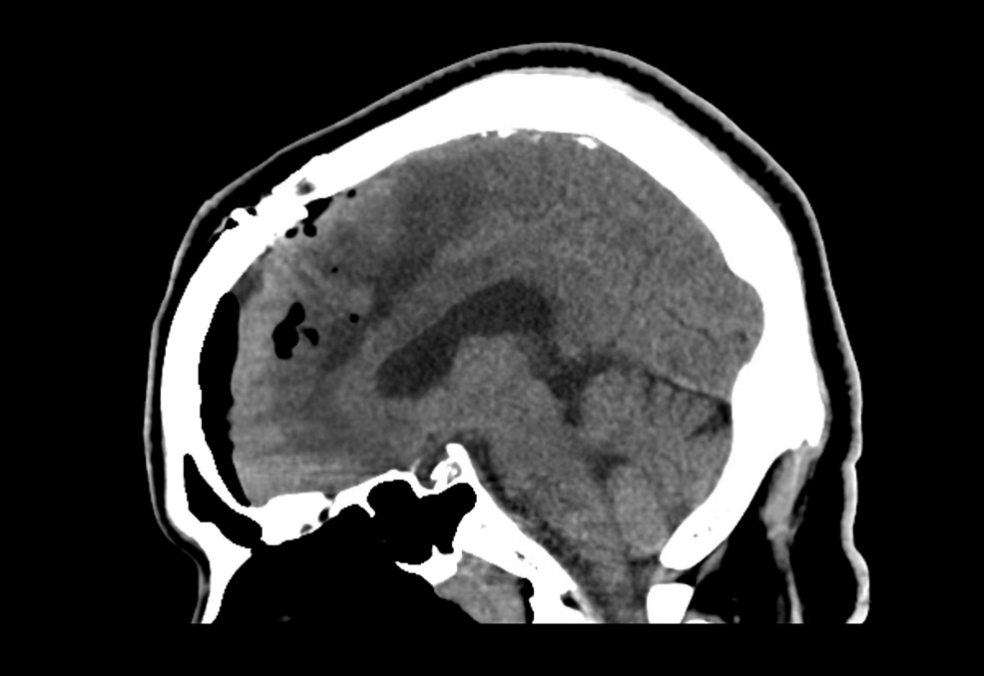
Immediate postoperative CT. CT, computed tomography
